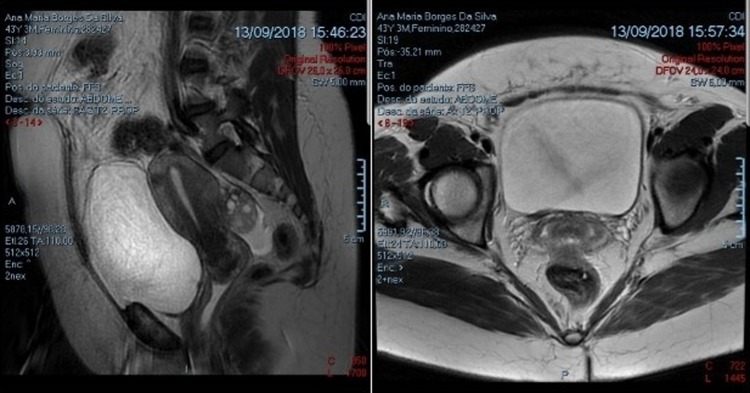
Follow-up magnetic resonance imaging 2 months after procedure showing no abnormalities.
